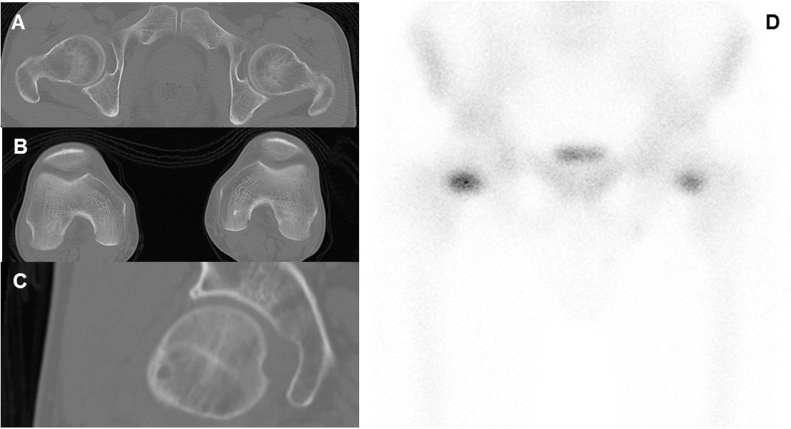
(A,B) Computed tomography axial planes indicate normal femoral neck anteversion on both hips, (C) multiplanar reconstruction shows herniation pit at lateral femoral head-neck junction on the right hip. (D) Bone scintigraphy scanning shows increased uptake in both femoral necks.
